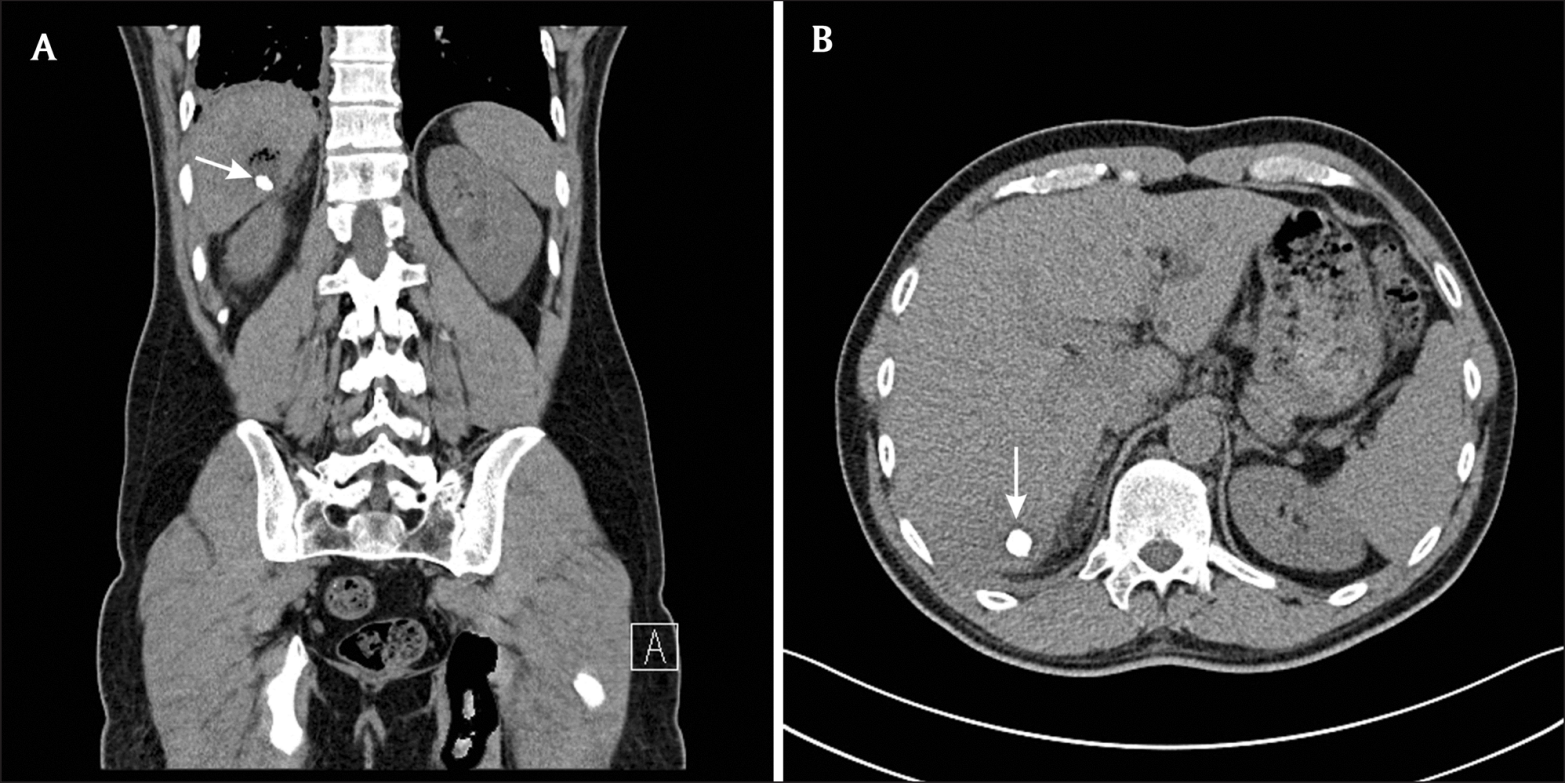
Coronal and axial reconstruction of the non-contrast enhanced CT-scan performed at readmission. Intrahepatic localization of the former seen appendicolith surrounded by a hypodense zone with air bubbles, indicating pus.
